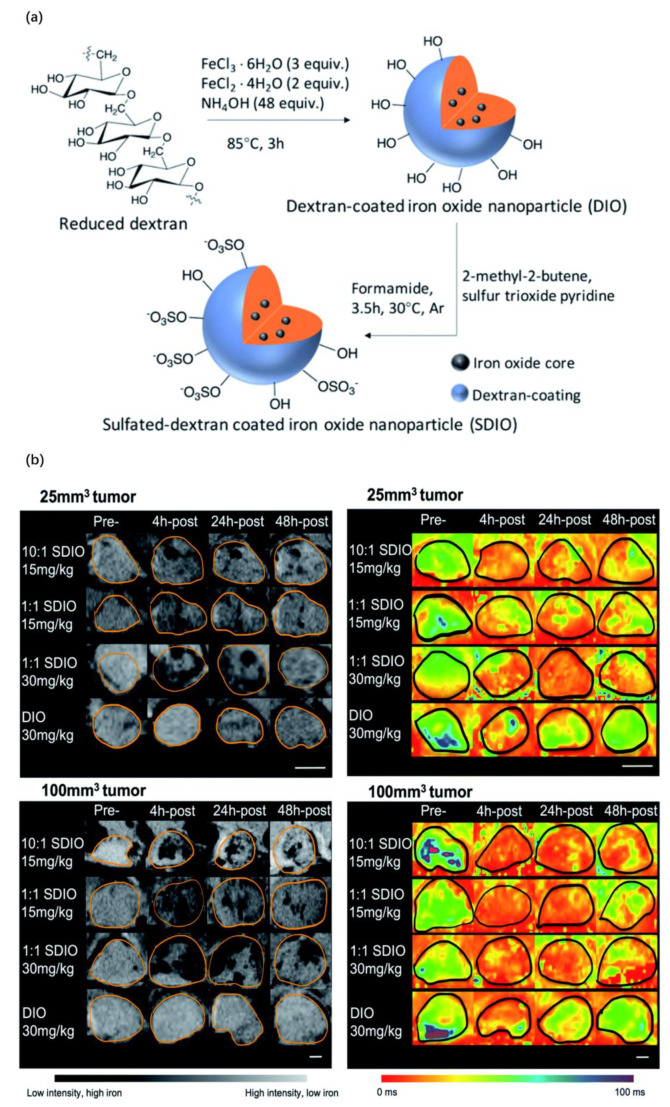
(a) Synthetic route of SDIO/DIO. (b) Representative zoomed in MR images and parametric T2* maps for 25 mm^3^ tumor and 100 mm^3^ tumor models injected with either 15 mg Fe kg^−1^ 10:1 SDIO, 15 mg Fe kg^−1^ 1:1 SDIO, 30 mg Fe kg^−1^ 1:1 SDIO, or 30 mg Fe kg^−1^ DIO at Pre-, 4 h-post, 24 h-post, or 48 h-post injection time point (tumor margins are outlined in orange) [23]. Copyright 2022, The Royal Society of Chemistry (Reprinted from Ref. [23] under the terms of the Creative Commons CC BY license).
